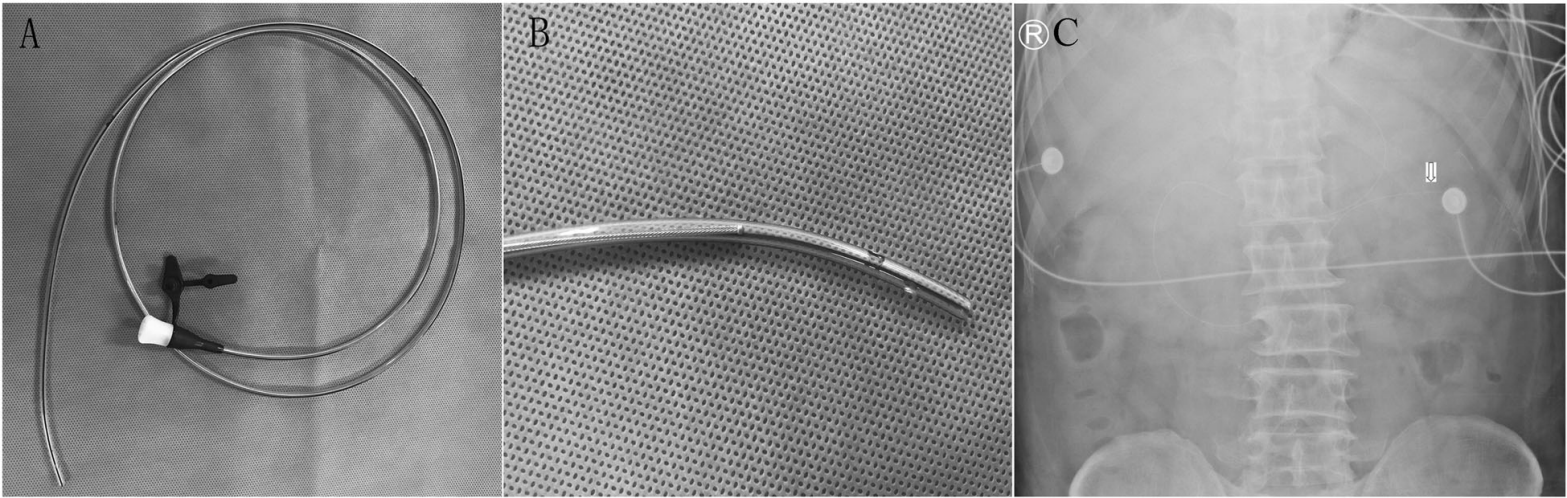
The 130-cm-long transpyloric tube with a guide wire (CH10-130, inner diameter 2.0–2.1 mm, Flocare, Nutricia Ltd., Wuxi, China) used in our unit (a). This Flocare tube has two side holes near its tip (b). Abdominal plain radiograph showed the tip of Flocare tube was positioned near the Treitz ligament (c)
